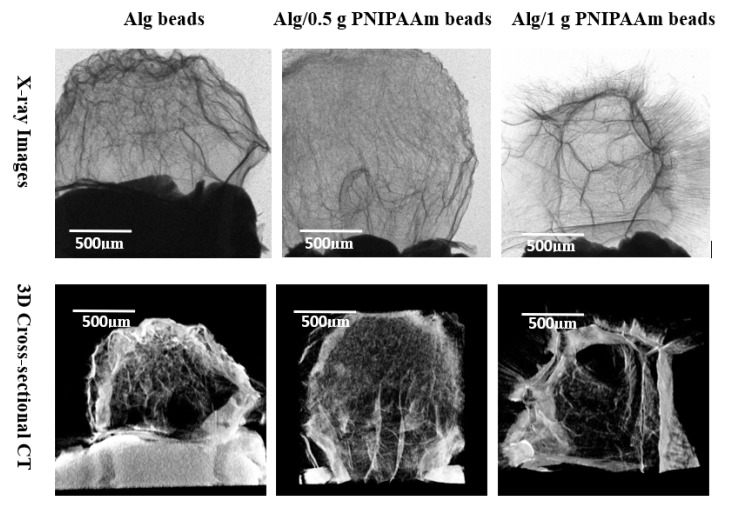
Both X-ray (2D) and cross-sectional CT (3D) images of Alg beads, Alg/0.5 g PNIPAAm beads and Alg/1 g PNIPAAm beads showing internal patches and fibrotic orientation leading to roughness. The surfaces of Alg/0.5 g PNIPAAm beads and Alg/1 g PNIPAAm beads exhibit clear fibrous structures. Alg beads have a typical surface of freeze-dried hydrogel pattern.
